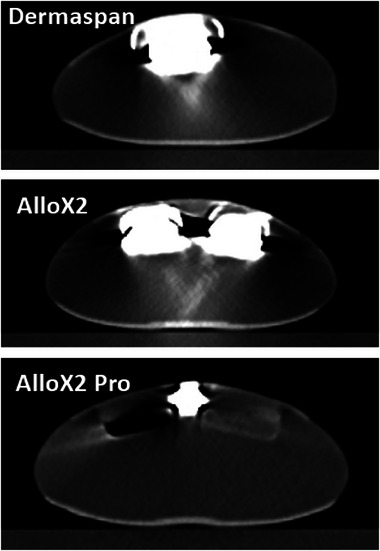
Comparison of iMAR CT scans of Dermaspan (top), AlloX2 (middle), and AlloX2 Pro (bottom).
